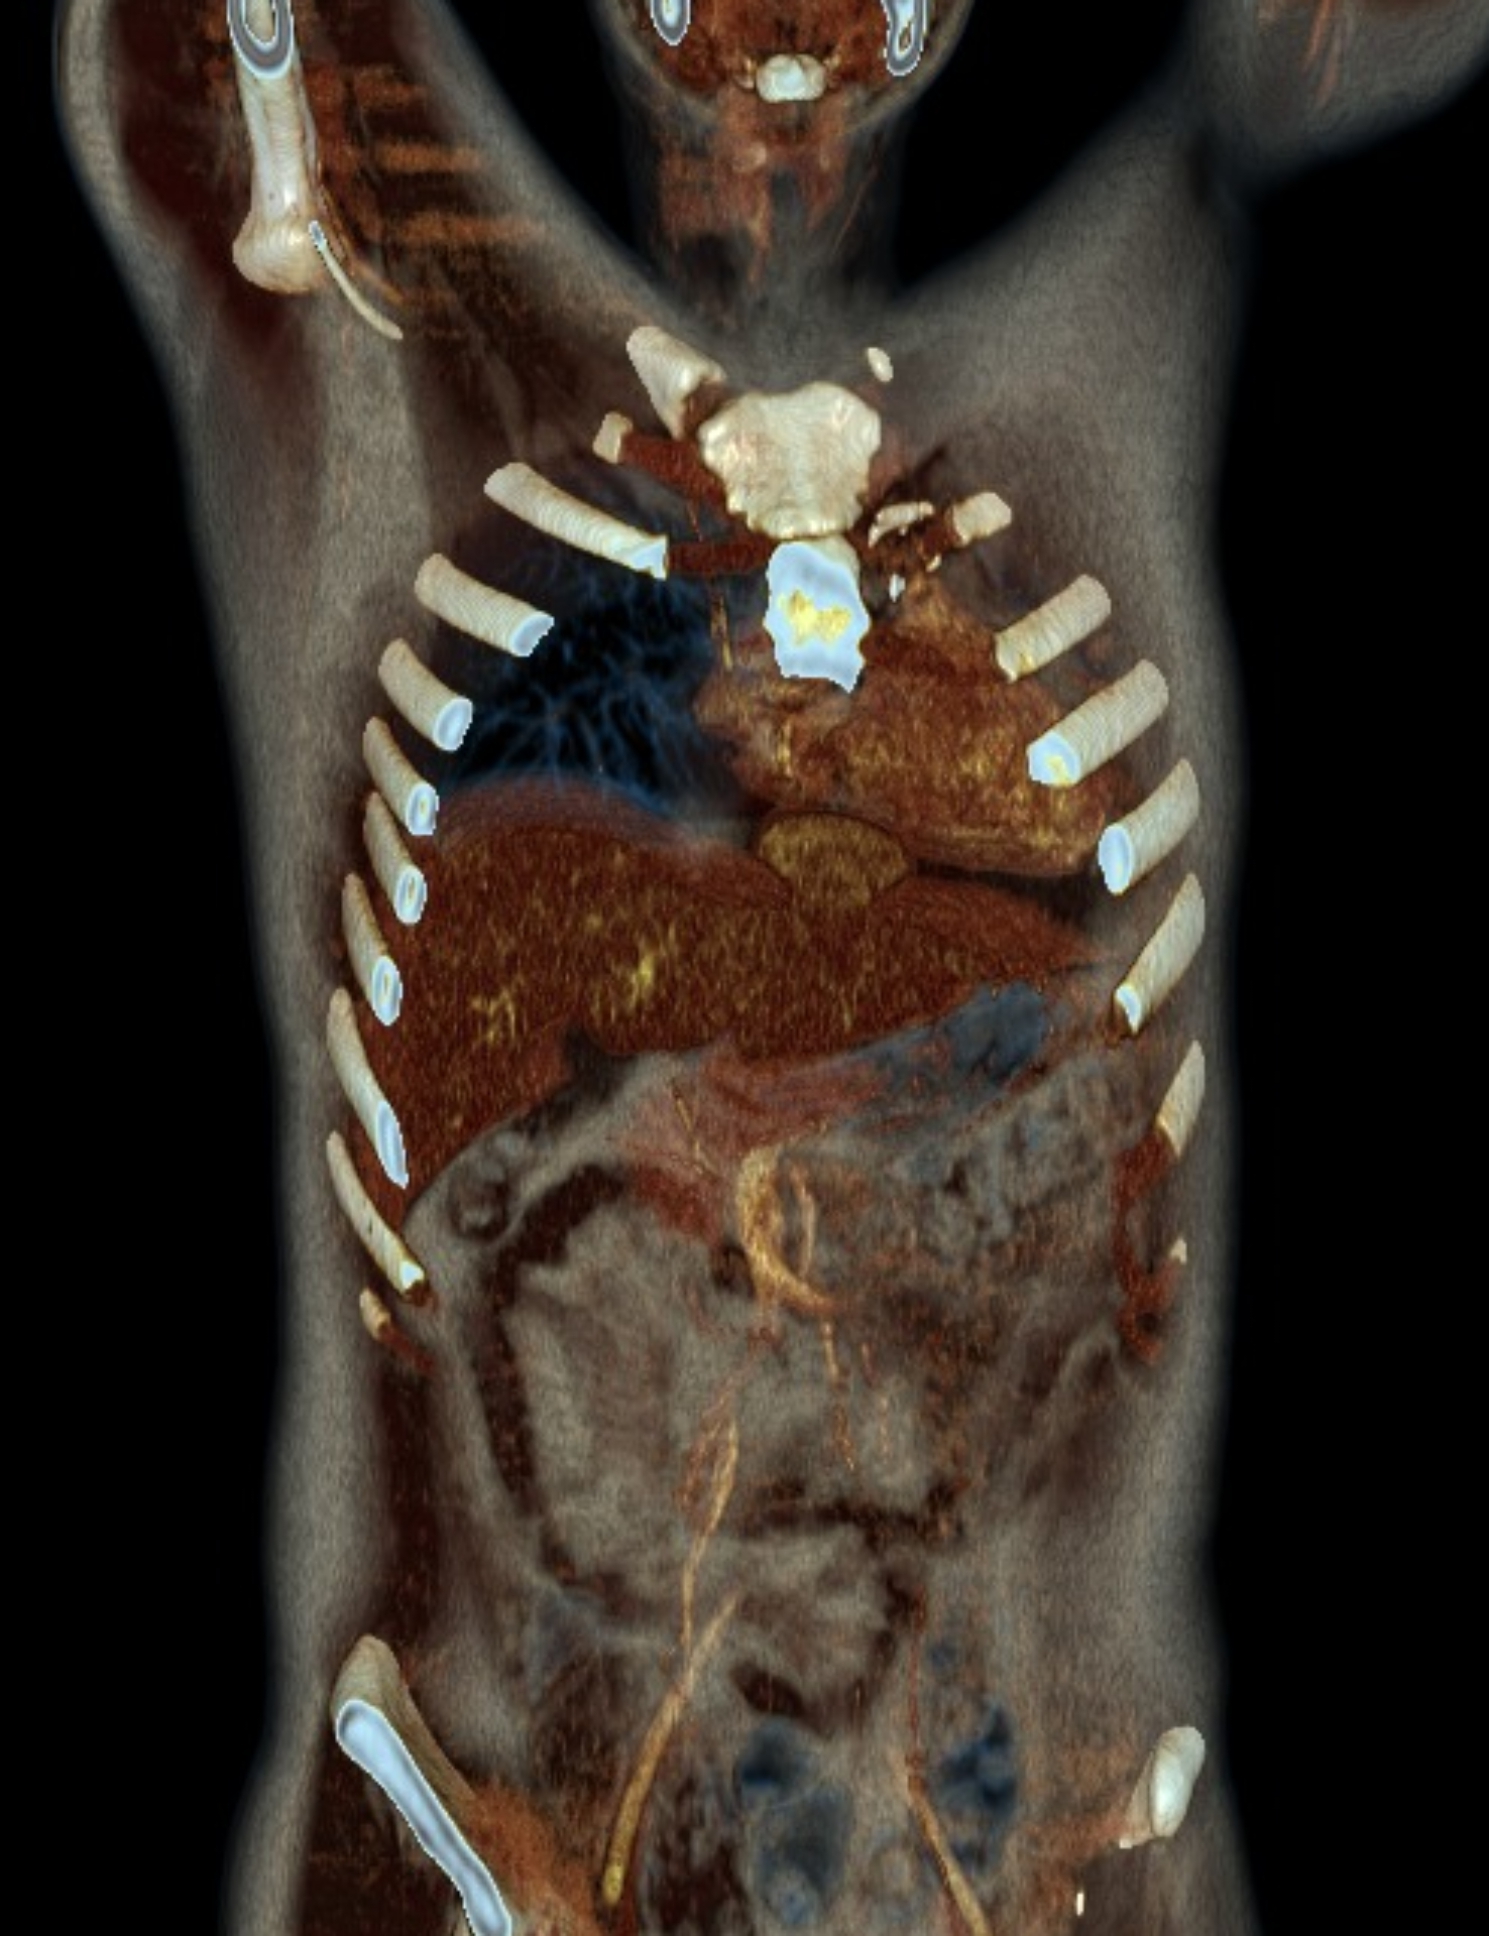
3D reconstruction of PET/CT scans with the main findings of liver herniation into the thoracic cavity
